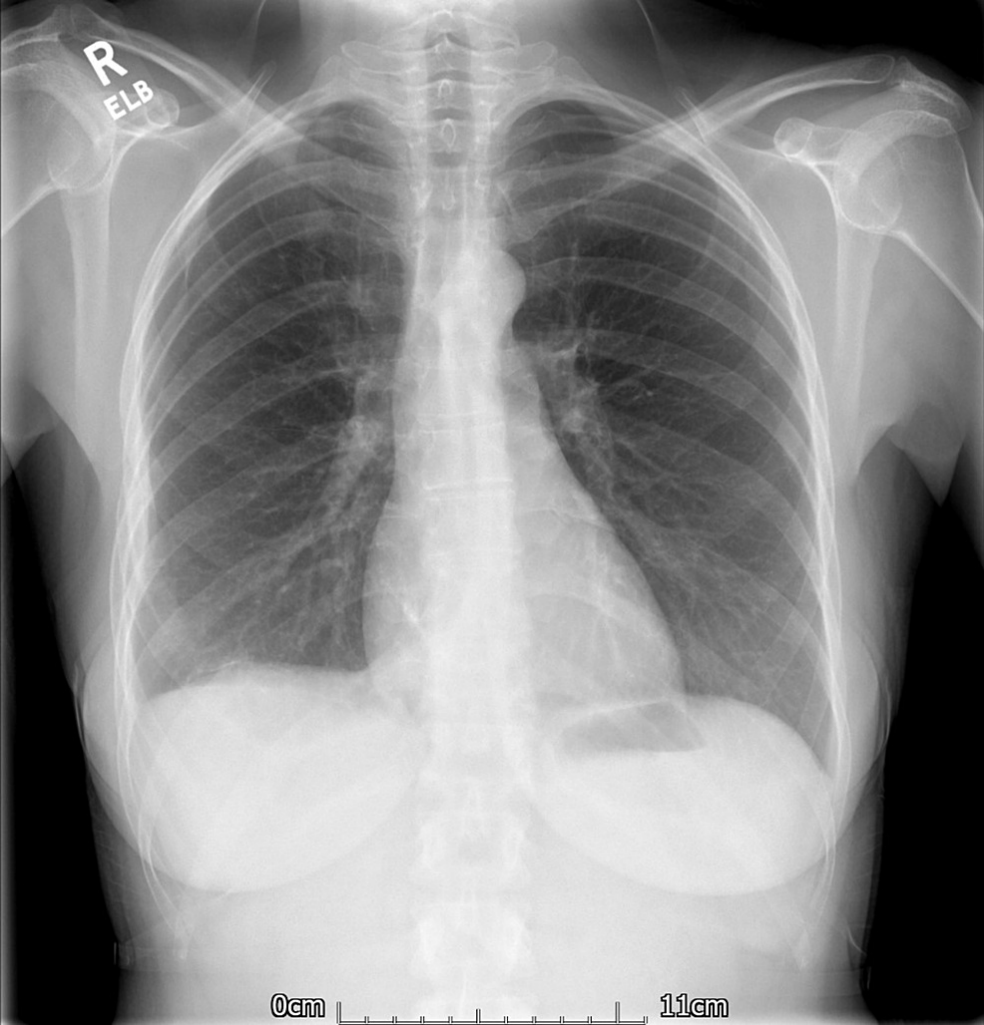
Posteroanterior (PA) chest X-ray, performed on the sixth post-operative day, demonstrating clear lung field bilaterally. No obvious masses, nodules, consolidation or collapse visible. No mediastinal shift.
